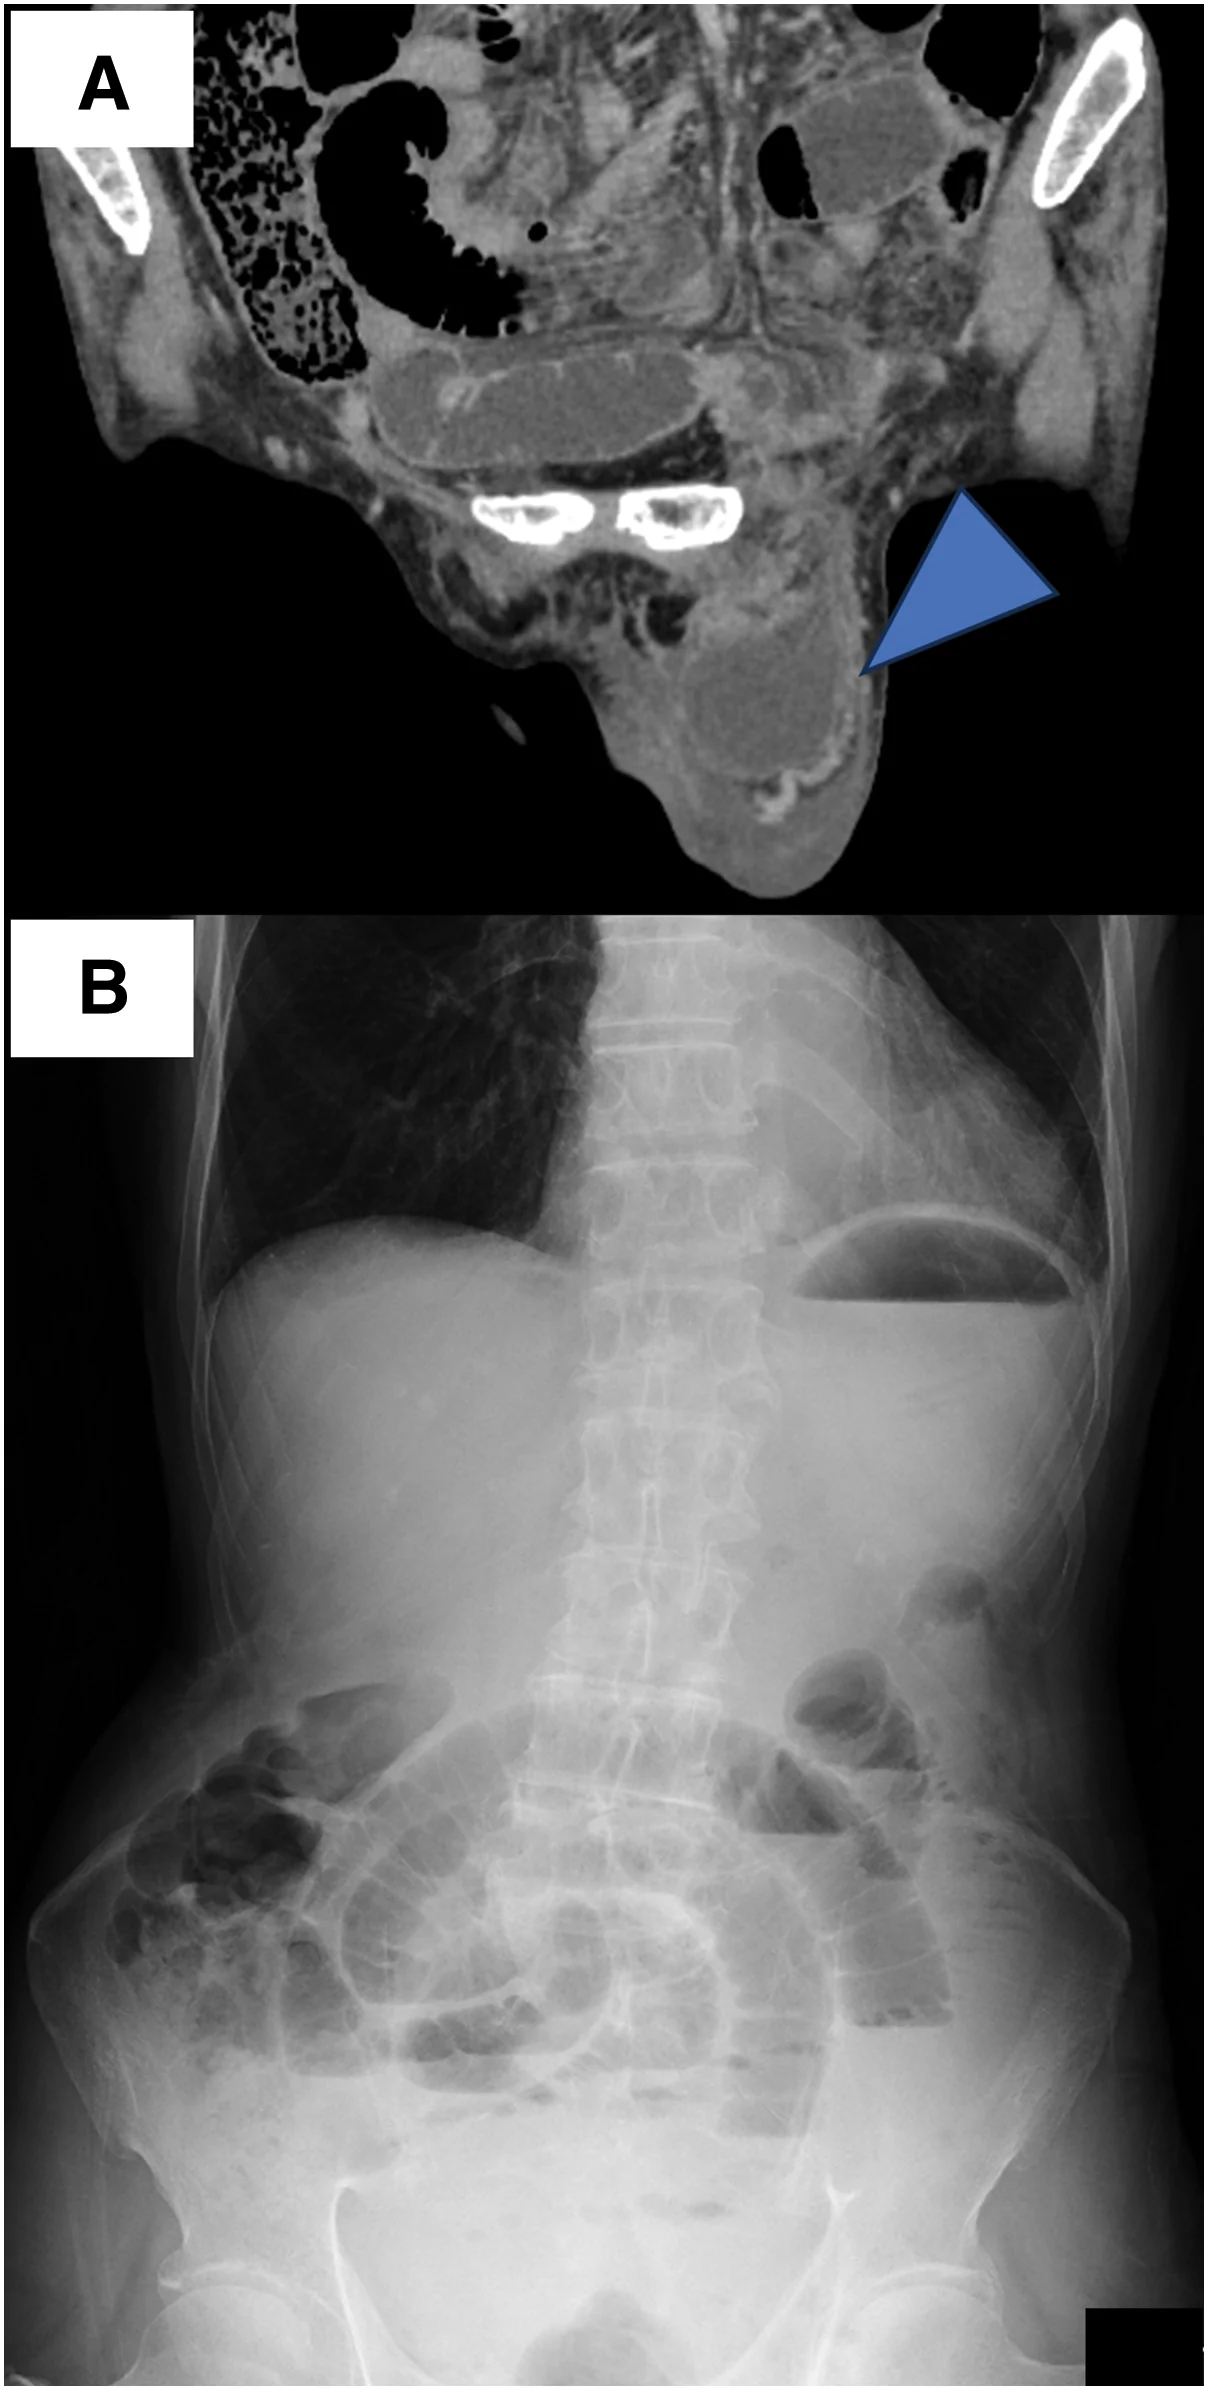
Post-reduction imaging. (A) Contrast-enhanced CT obtained immediately after reduction demonstrating reduction of the herniated bowel without obvious signs of ischemia or persistent incarceration. The residual hernia sac contained only ascites (arrowhead). (B) Abdominal radiograph obtained approximately 8 hours after reduction showing the persistent dilatation of the small bowel with multiple air-fluid levels.
